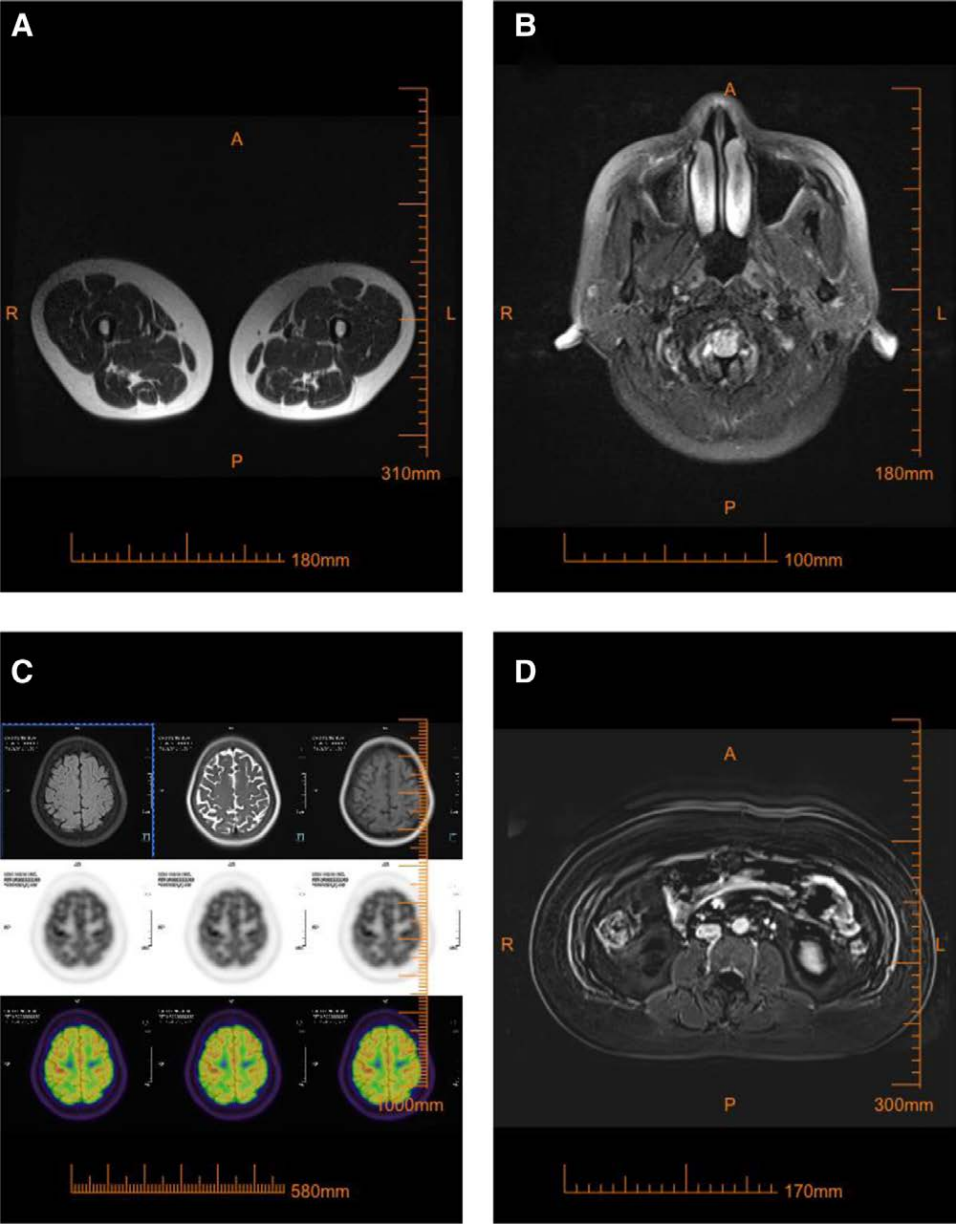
Whole-body PET-MRI. (a) Sagittal. (b) Axial. (c, d) Coronal. PET-MRI = Positron emission tomography–magnetic resonance imaging.
